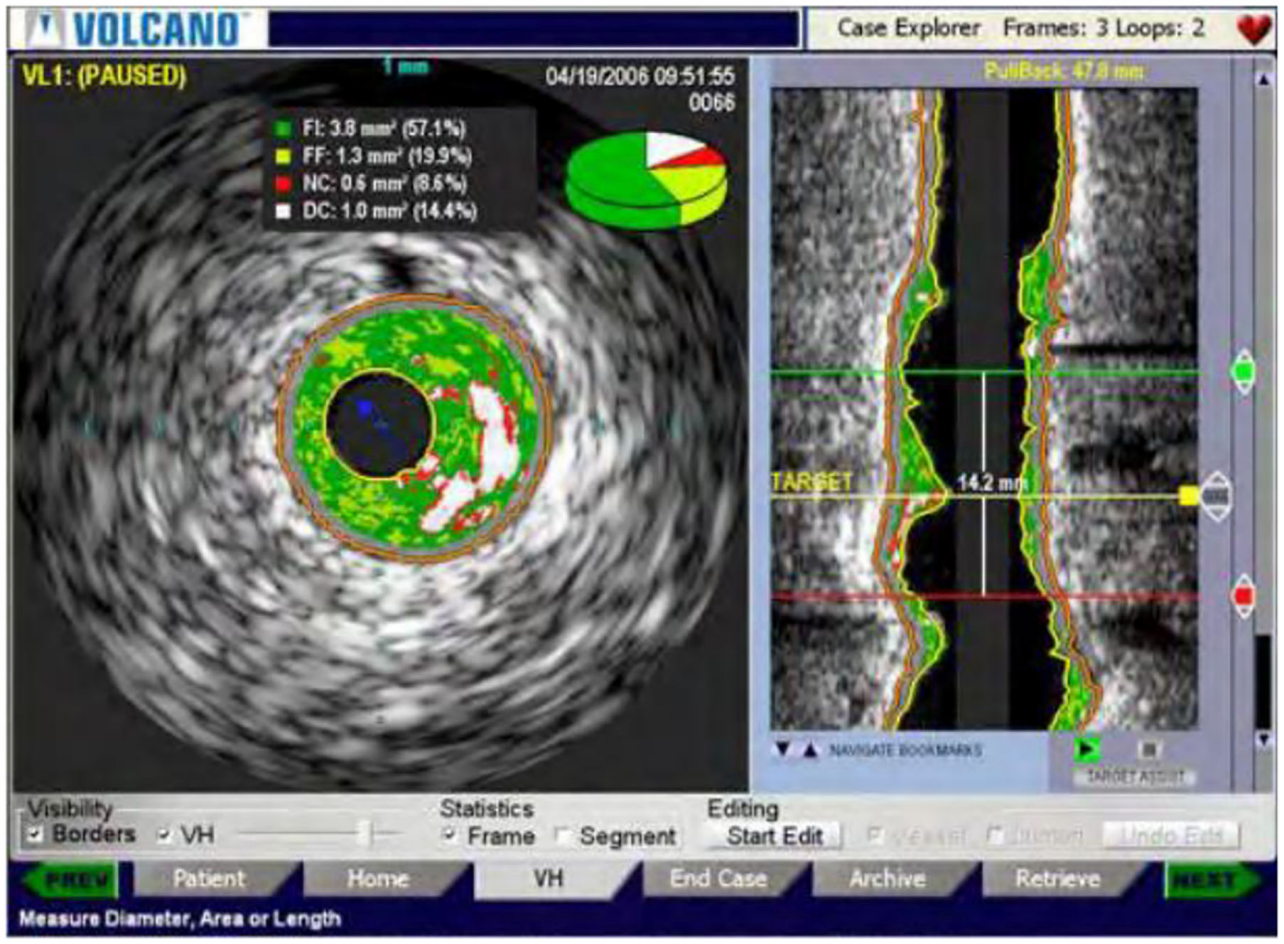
VH-IVUS image of a new type of intravascular ultrasound.
